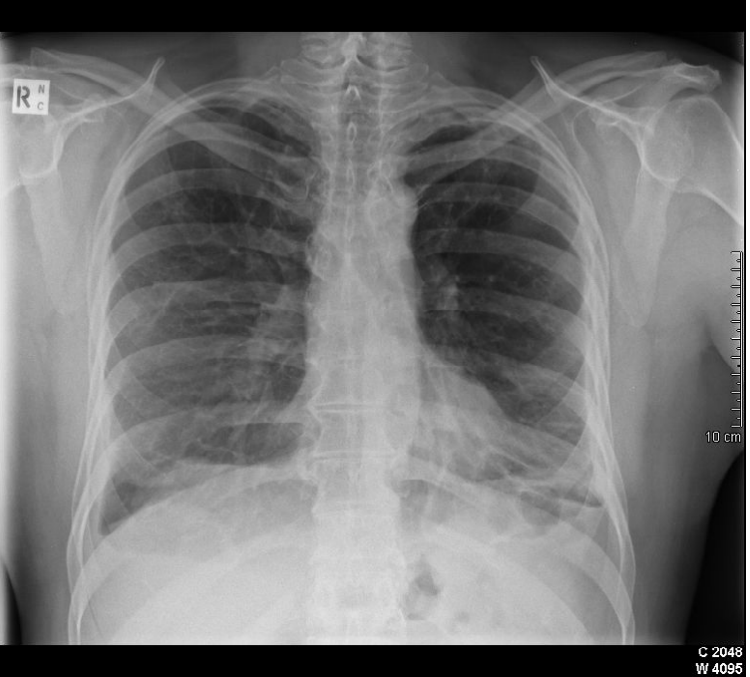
Postero-anterior chest radiograph demonstrating asbestos-related diffuse pleural thickening.
